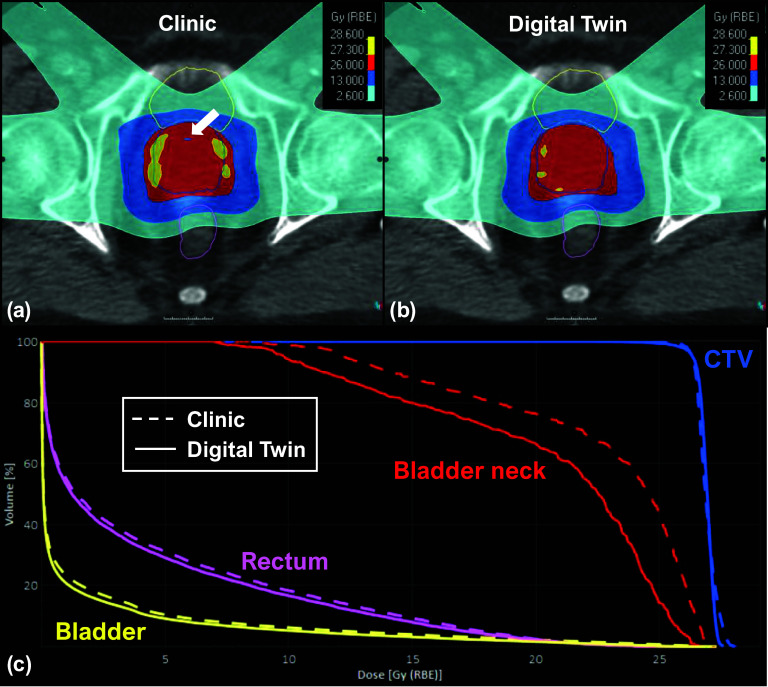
Dosimetry comparisons for Patient 3 between the clinical and DT-based plans. The top row displays the dose distributions in color wash for the (a) clinical plan and (b) DT-based plan, overlaid with transversal CT images and the contours of CTV (blue lines), bladder (yellow lines), and rectum (magenta lines). The bottom row (c) displays the dose-volume histogram (DVH) for CTV and OAR structures, where the dashed and solid lines represent the clinical plan and DT-based plan. The white arrow indicates the cold spot location in CTV.
